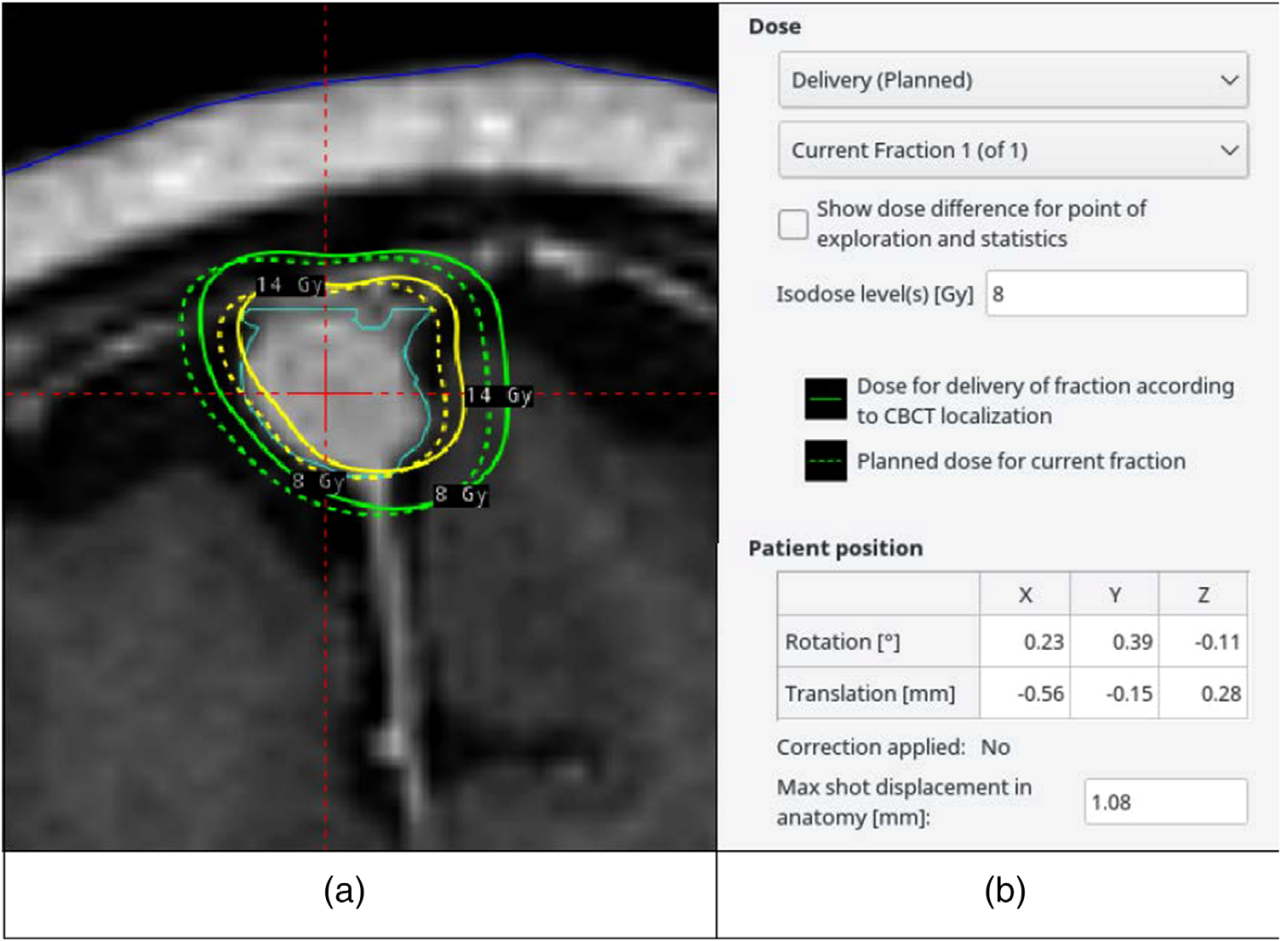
A snapshot from the Leksell GammaPlan treatment planning demonstrates both the shifts and dose distribution differences between the planned treatment (based on stereotactic frame) and the CBCT‐based one. CBCT, cone beam computed tomography
